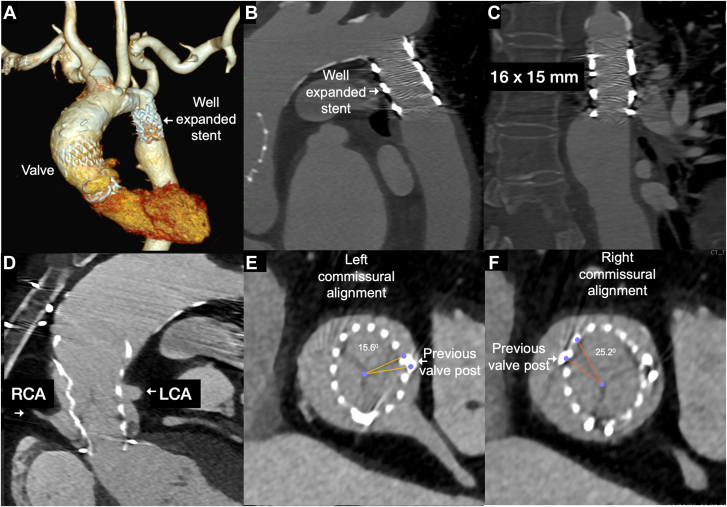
Postprocedural computed tomography. (A-C) Fully expanded coarctation stent (arrow), no pseudoaneurysm and well flowing left coronary artery (LCA, arrow) and right coronary artery (RCA, arrow) through the valve frame. The commissural alignment to the previous valve posts (arrow) shows normal alignment of left (E) and mild misalignment to right (F).
